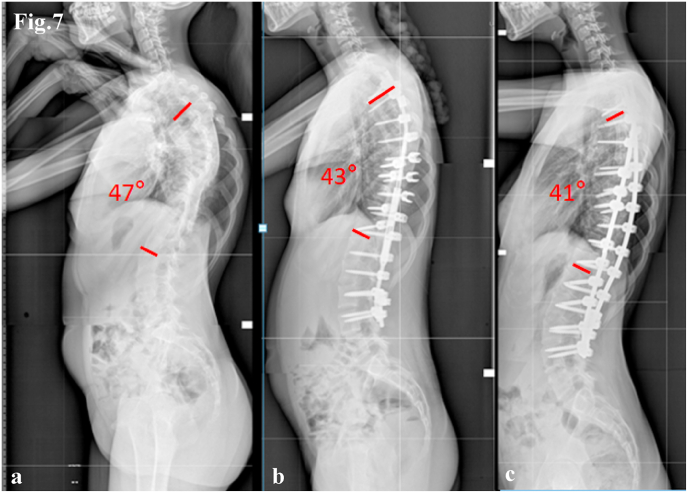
Case 2. Lateral radiographs show: (a) preoperative curve, (b) correction with MCGR, (c) final correction with definitive titanium/cobalt–chrome rods.
